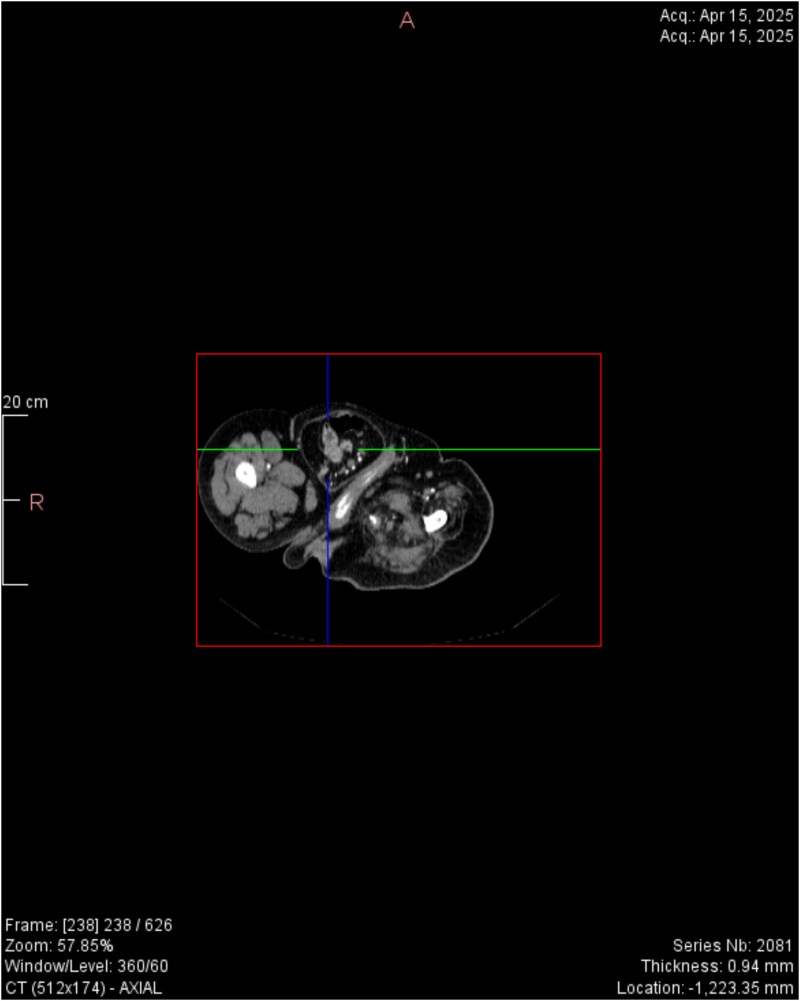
Horizontal CT view inguinal hernial sac with pancreatic head and D2 and D3 segment of duodenum.
